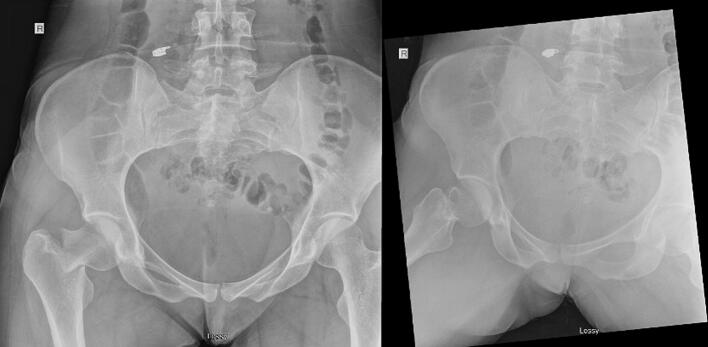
Anterior-posterior (AP) and lateral radiographs demonstrating a right displaced, transcervical femoral neck fracture.
